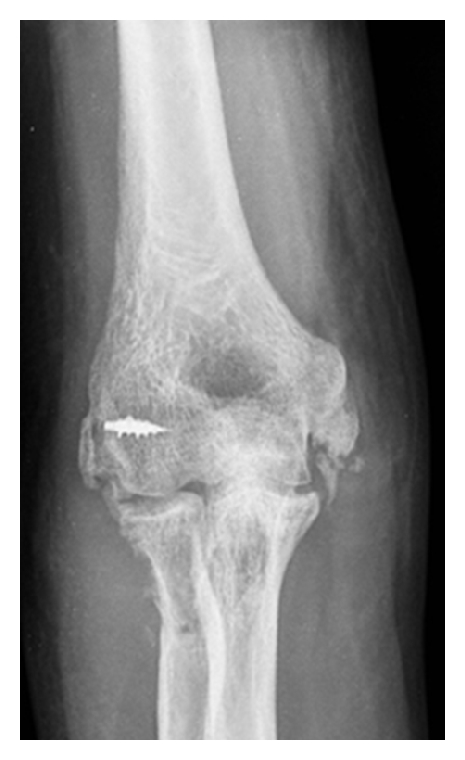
The figure shows an X-ray of a right elbow, 50 days after an LCL repair. The ROM was 80° in extension and 110° in flexion.
